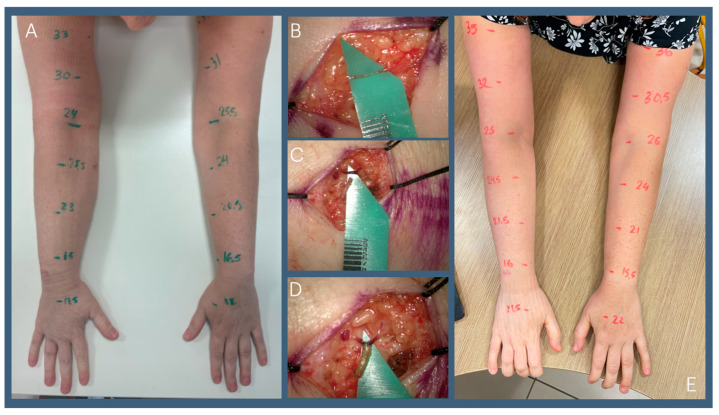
Forty-two-year-old patient who suffers from Rt BCRL. (A) Pre-op; (B) 0.3 mm lymphatic vessel in the distal arm; (C) 0.6 mm vein was found (pre-operative ultrasound assisted in locating lymph vessel and adjacent vein); (D) side-to-end anastomosis of lymph vessel to vein using 11-00 nylon sutures; (E) 3-months post-op shows improvement in limb circumferences.
